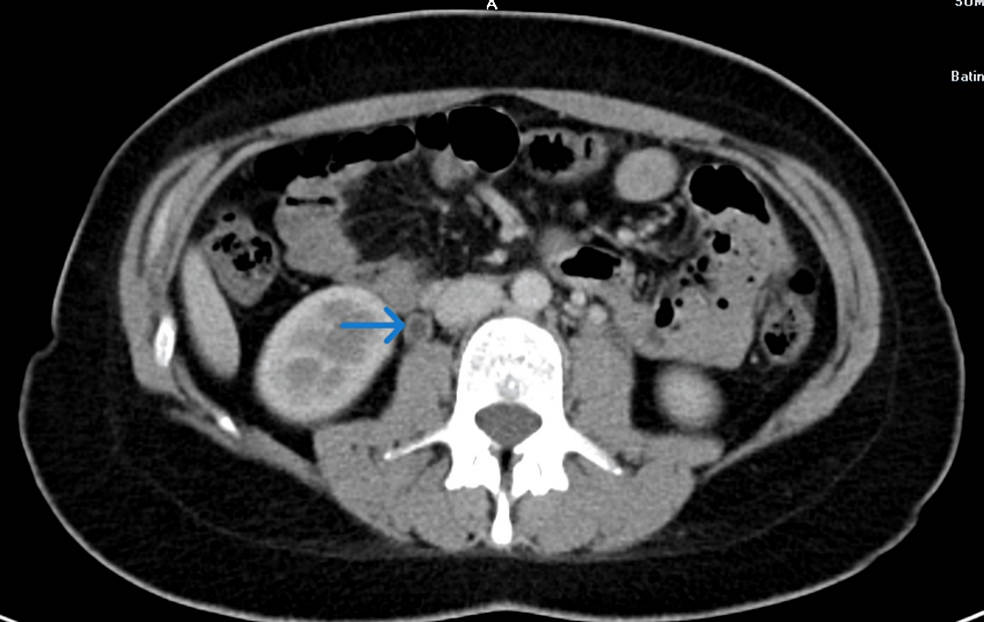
Axial Contrast-Enhanced CT View of Thrombosis
